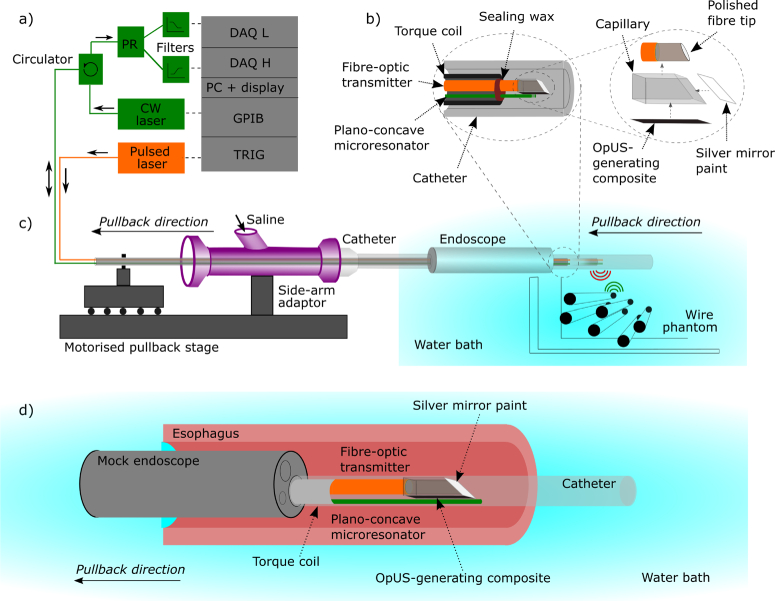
System for all-optical lateral ultrasound imaging through
